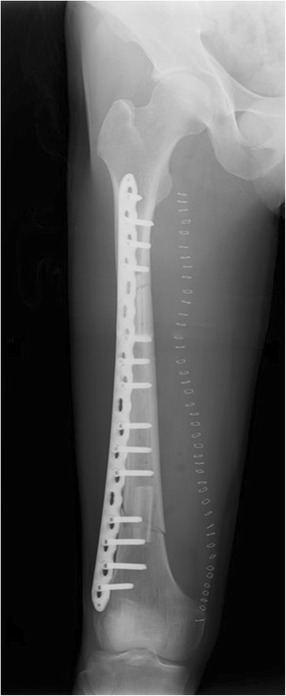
Postoperative radiography after the femur reconstruction
